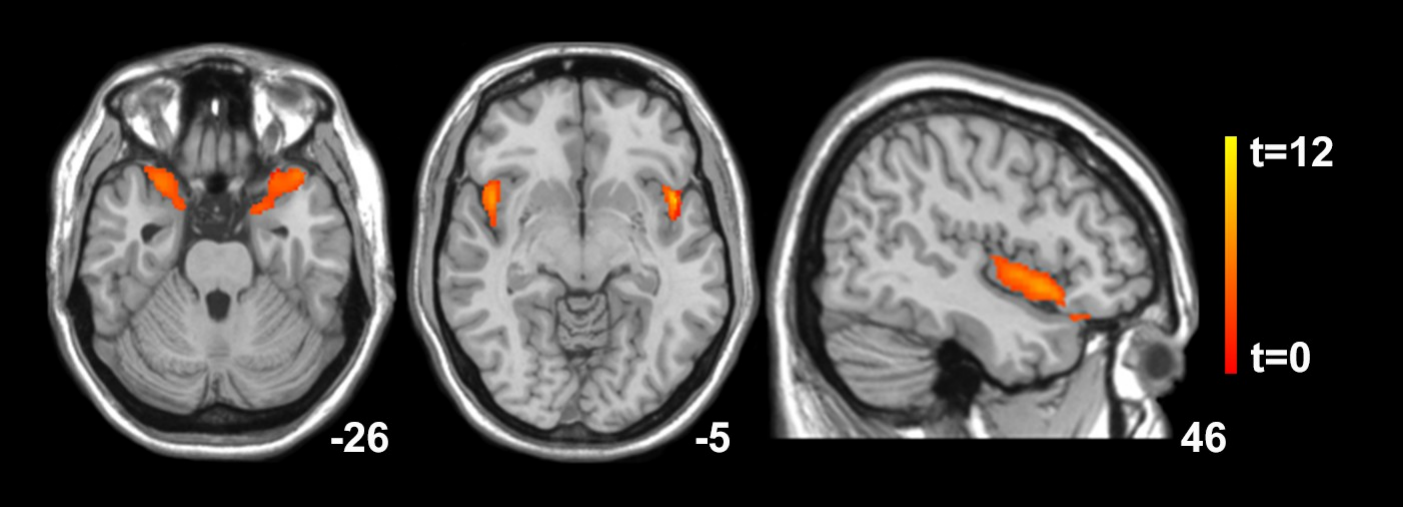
Statistical parametric mapping (*t*) intensity projection maps showing areas of significant decreases of grey matter volume (color code, yellow to orange) in a cohort of people with multiple sclerosis compared to healthy control subjects at baseline rendered on to a stereotactically normalized MRI scan in MNI space (z = -26 and -5; x = 46). The right side of the image corresponds to the right side of the brain.
